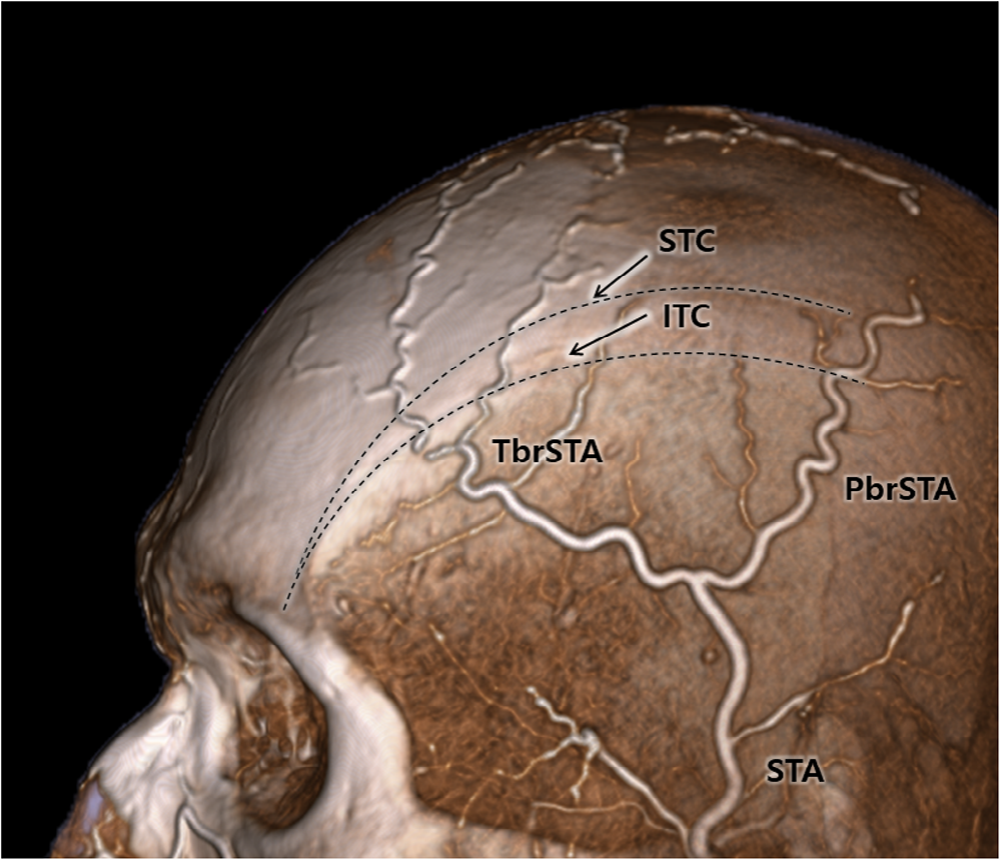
Computed tomography angiography three‐dimensional reconstruction showing the superficial temporal artery (STA) with parietal (PbrSTA) and frontal branches (TbrSTA). The superior temporal crest (STC) and inferior temporal crest (ITC) are guided as dotted line.
